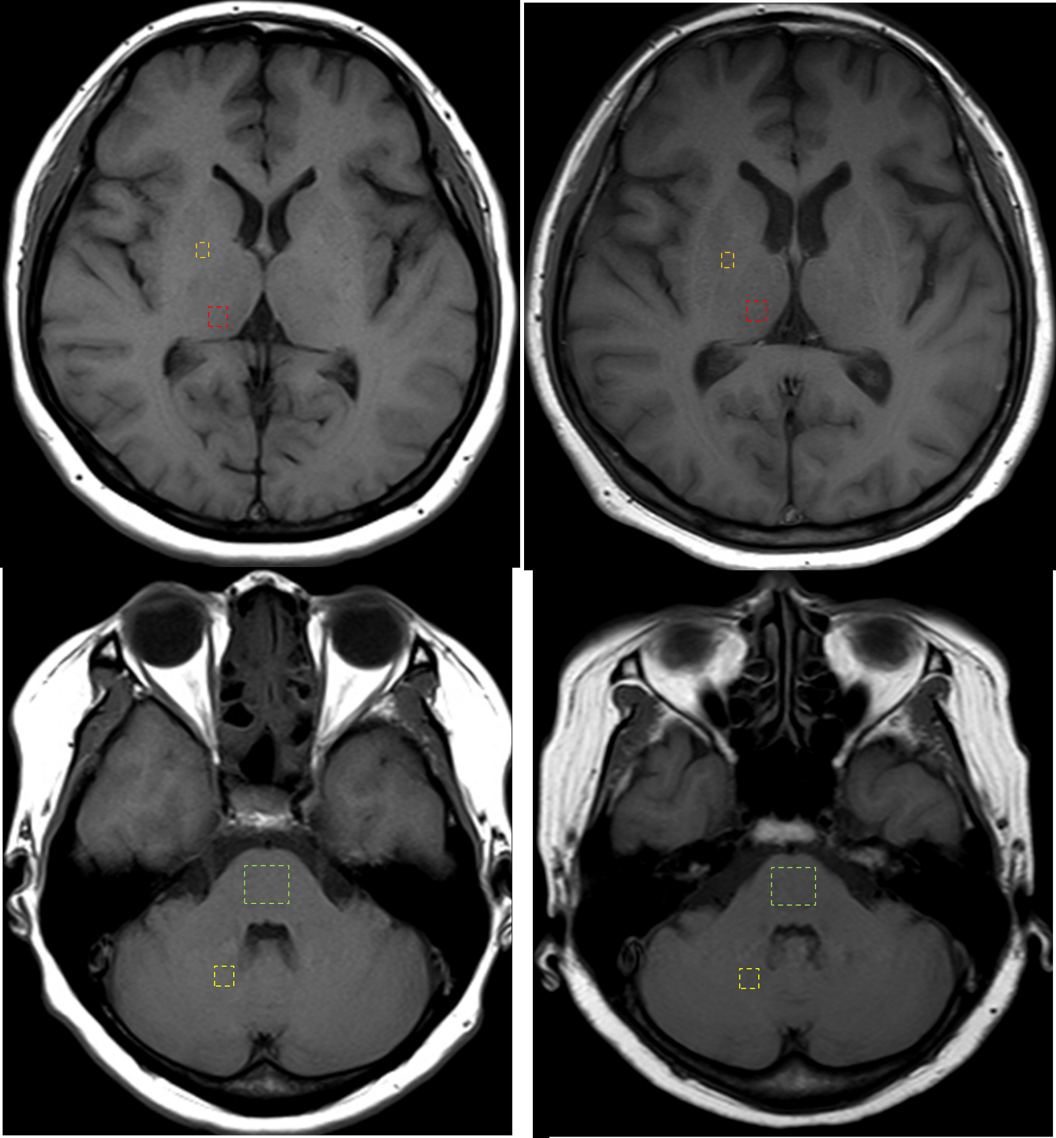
Axial T1-weighted MR images at the level of the basal ganglia and dentate nucleus. The regions of interest used in the quantification of signal intensity are shown as dashed lines for the globus pallidus (GP, orange), thalamus (Th, red), dentate nucleus (DN, yellow), and pons (P, green). (upper row) GP and Th in the first examination and after 21 administrations of macrocyclic ionic MR contrast agent. (lower row) DN and P in the first examination and after 21 administrations of macrocyclic ionic MR contrast agent.
